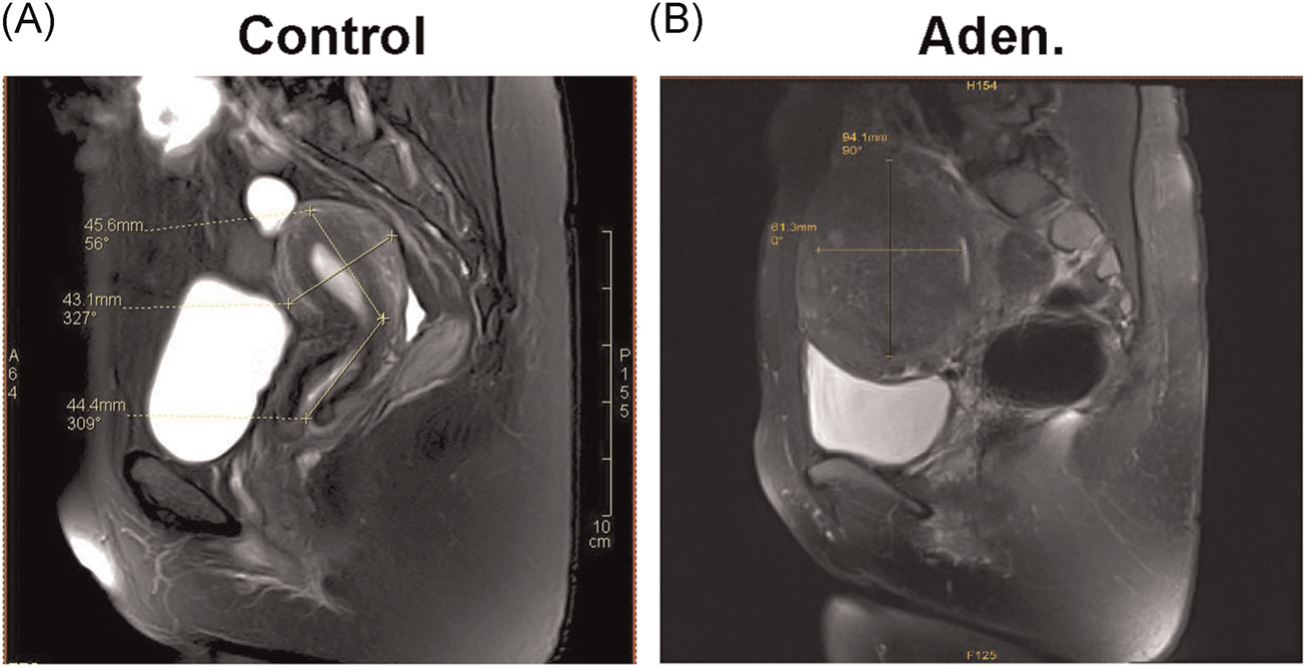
Representative pelvic MRI from the control and adenomyosis patients. (A) In a control patient (control), the MRI picture shows the normal uterine, with dimensions of a 45.6‐mm length and 43.1‐mm width crossing the whole uterus. (B) In an adenomyosis patient (Aden.), the MRI scan shows typical focal adenomyosis, with a 40.6‐mm length and 56.9‐mm width of pathological foci located in the uterine junctional zone (indicated with a red star). MRI, magnetic resonance imaging
